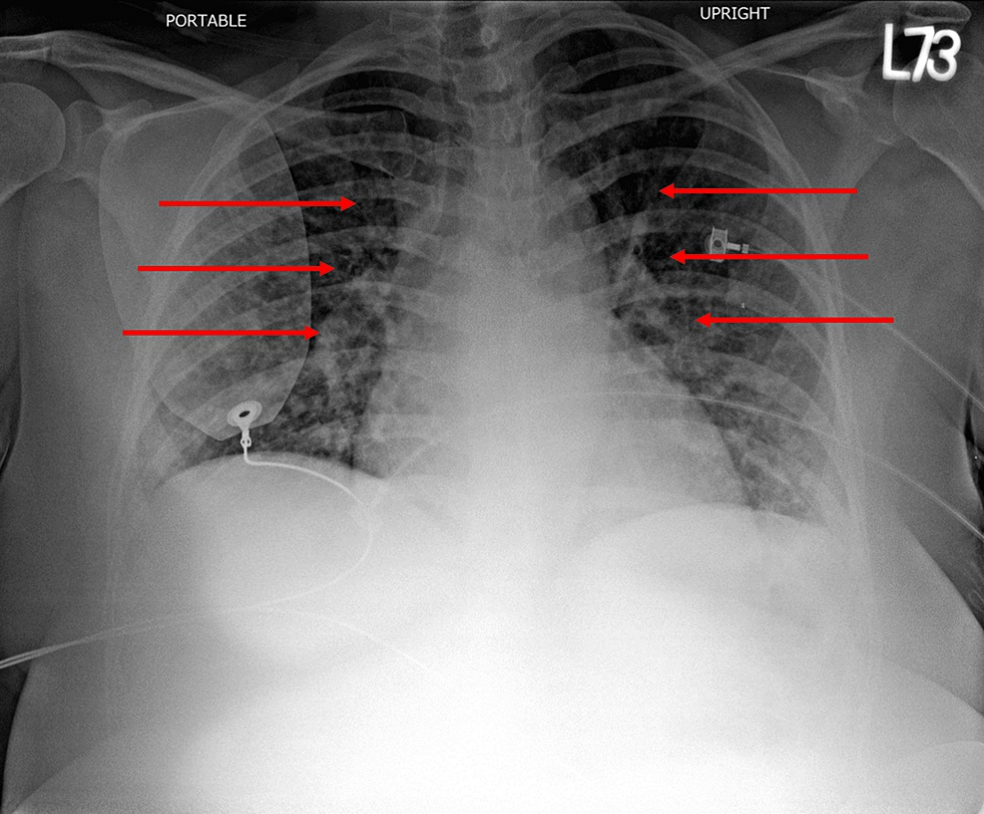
Chest X-ray showing enlarged cardiac silhouette with interstitial infiltrates.
